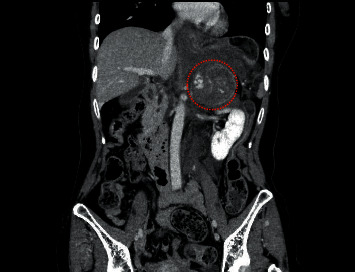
Computed tomography findings on admission. Contrast-enhanced coronal abdominal computed tomography showed a left 5.7 cm adrenal mass (red dots) and a large retroperitoneal hemorrhage.
